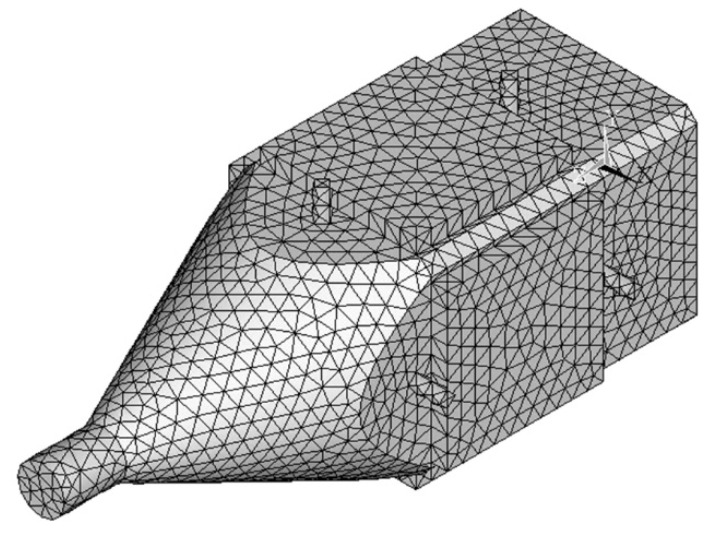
The FEM model of the proposed ultrasonic motor built in ANSYS.
